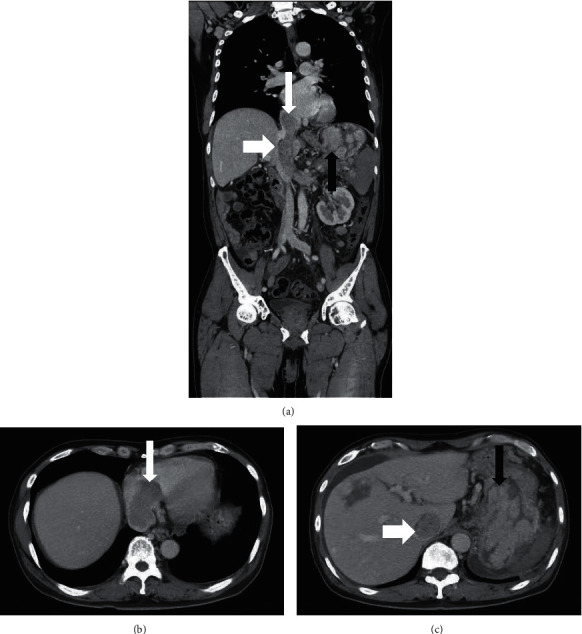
Enhanced CT of venous phase images of the patient. (a) CT enhanced coronal section with three-dimensional reconstruction. (b, c) CT enhanced transverse sections. The long white arrow in (a) and (b) indicates right atrium cancer thrombus, the short white arrow in (a) and (c) indicates cancer thrombus in the inferior vena cava, and the long black arrow in (a) and (c) indicates gastric metastasis.
